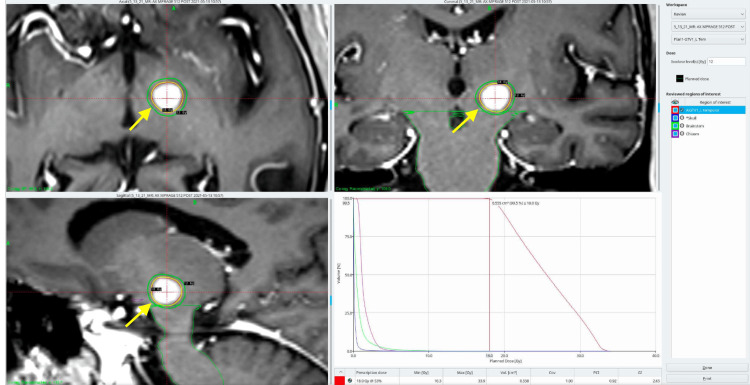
MRI depicting the tumour location with contours and the DVH The figure depicts the tumour's exact location in the three dimensions, and the contours are marked with a yellow arrow. A dose-volume histogram is also presented, demonstrating the doses to the various volumes of the target lesion and the organs at risk. Figure Credit: Martin Tom
